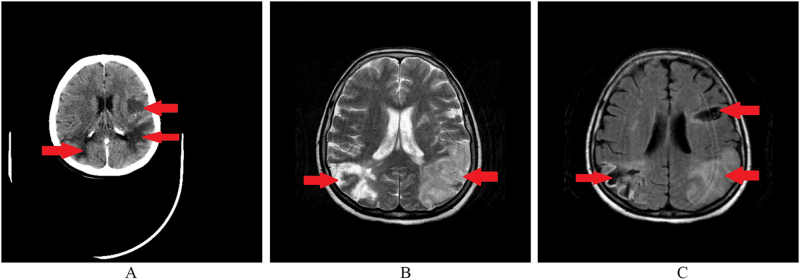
(A) CT scan, axial section. Arrows show several hypodense infarction-like lesions in the cerebral hemispheres. (B) T2 MRI, axial section. Arrows show hyperintense bilateral endemic lesions located in the cerebral hemispheres. (C) FLAIR MRI, axial section. Arrows show hypointense bilateral endemic lesions in the cerebral hemispheres.
